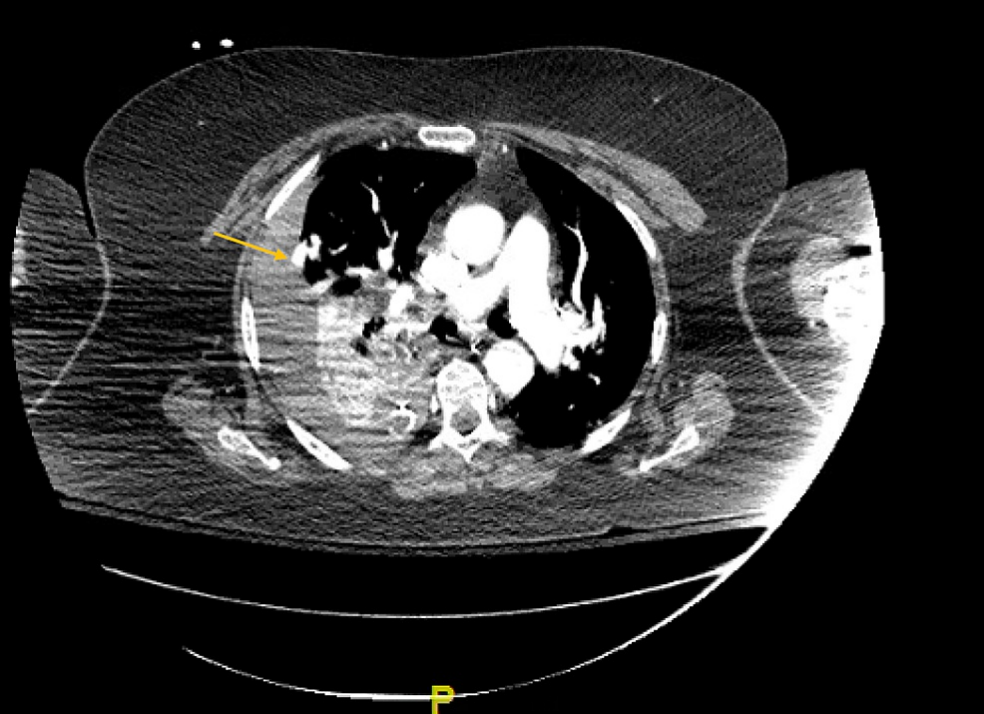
CT angiography demonstrating the arteriovenous malformation at the periphery of the right middle lobe (arrow). Large hemothorax can also be noted.
